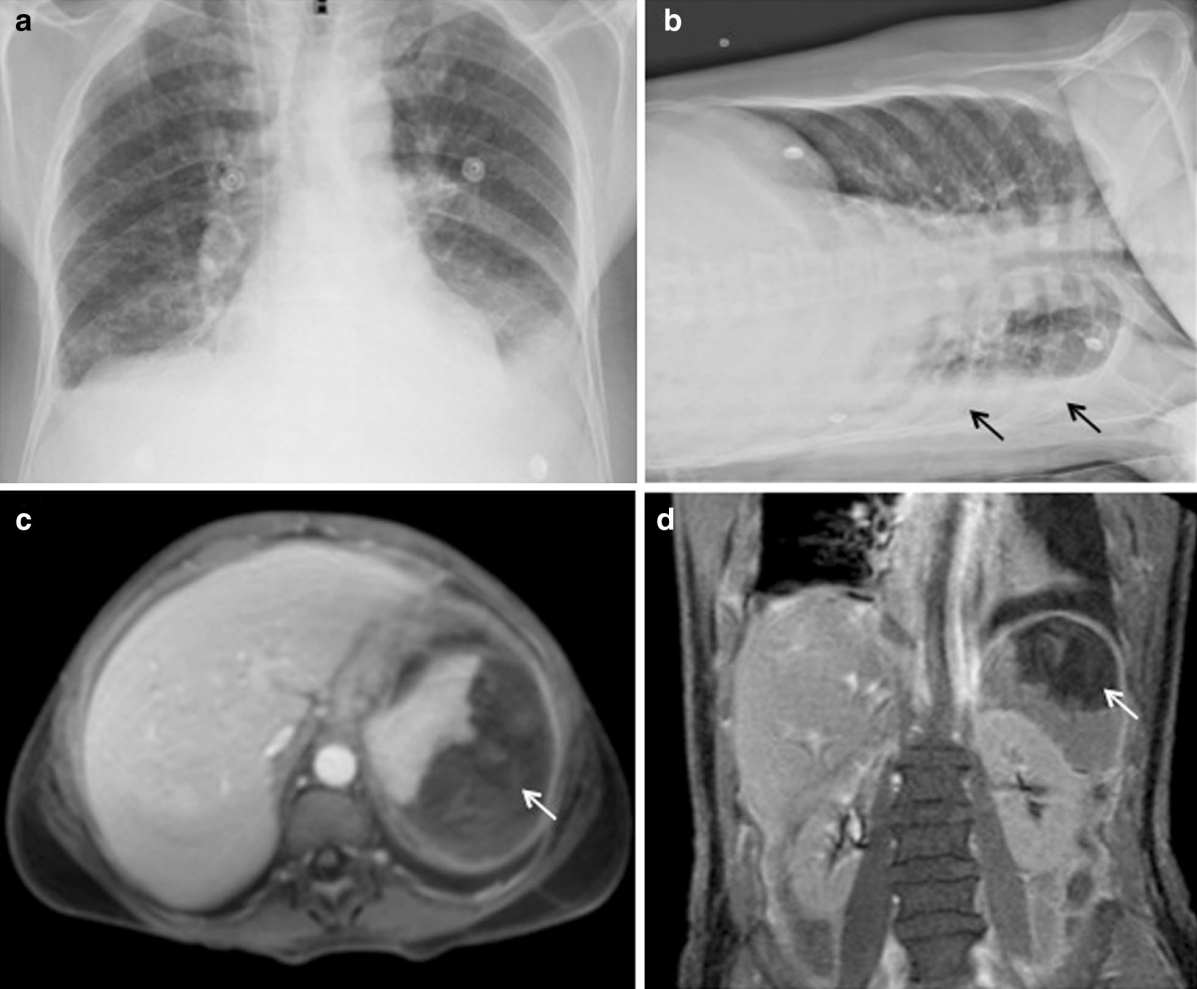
Radiologic findings of a man, 60 years of age, presenting severe vivax malaria. a Chest radiograph (PA view) showing interstitial opacities in both lungs compatible with pulmonary congestion. Obliteration of the costphrenic angles due to small bilateral pleural effusion can also be observed. b In the left lateral decubitus the pleural effusion is seen as fluid layering along the rib cage (*black arrows*). c Abdominal magnetic resonance imaging (T1-weighted axial image) reveals spontaneous sub-capsular splenic haematoma (*white arrow*). d The splenic haematoma is shown as T1-weighted coronal MRI image (*white arrow*)
